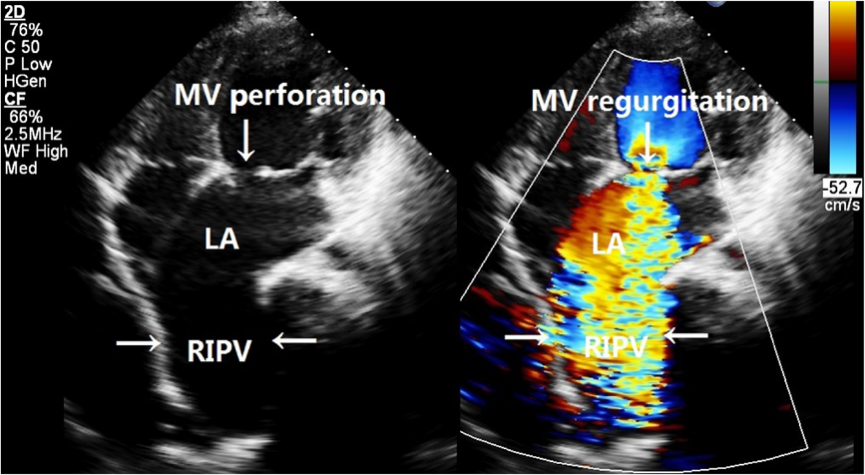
Echocardiographic images in split-screen mode revealing two-dimensional echocardiography and color Doppler simultaneously. Images showing high-velocity turbulent jet caused by perforation of the mitral valve filling in the RIPV. LA, left atrium; MV, mitral valve; RIPV, right inferior pulmonary vein.
